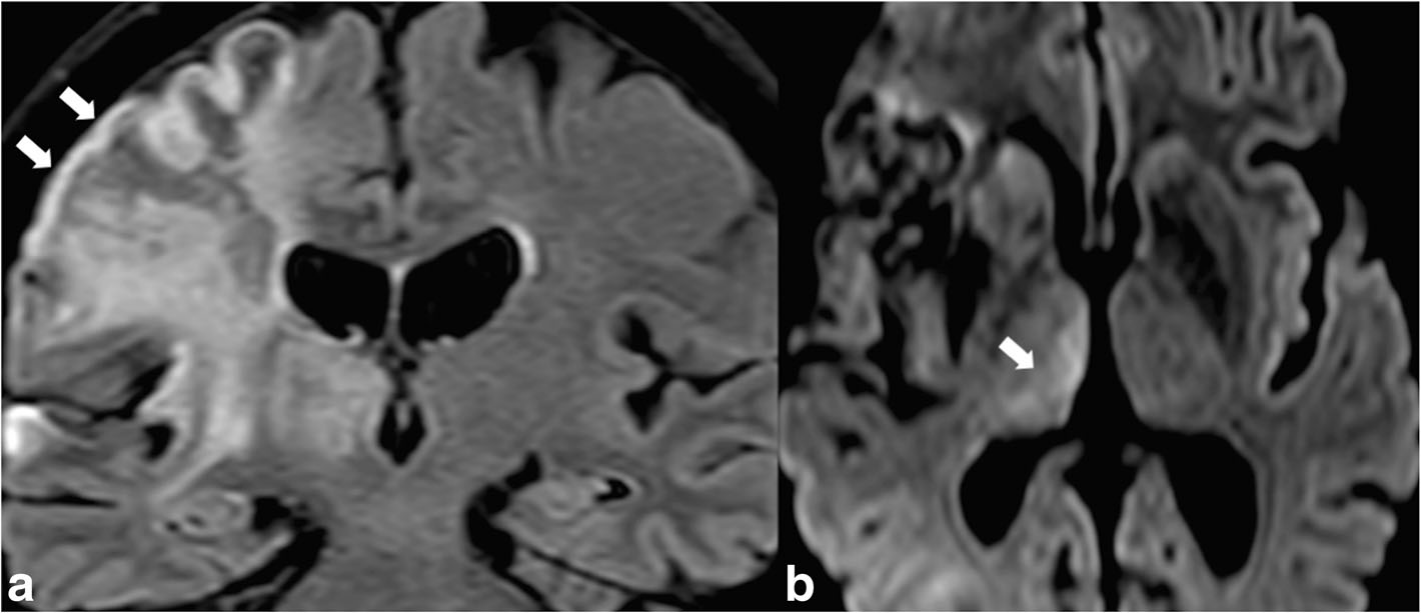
FLAIR hyperintensity in the right temporal and insular regions (arrows in a) reflecting brain ischaemia. An epileptic seizure ensued due to cortical involvement, which in turn resulted in high signal intensity on DWI b1000 in the right thalamus (arrow in b) as a consequence
